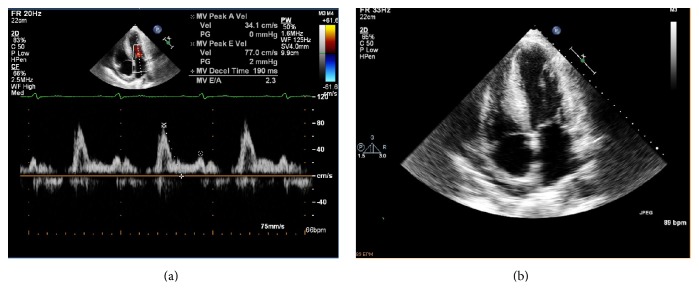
(a) At baseline, the transthoracic echocardiogram demonstrates moderately depressed LV function (LVEF-40%) with significant concentric and septal LV hypertrophy (LV mass 367 grams, IVSd-2.1 cm, PWT-1.5 cm, RWT-0.67). (b) There is further evidence of elevated filling pressures. There is left atrial enlargement (LA dimension-4.6 cm), mildly elevated estimated right ventricular systolic pressure (RVSP~36 mmHg), and restrictive filling on mitral inflow Doppler (E/A-2.3, deceleration time 190 milliseconds).
